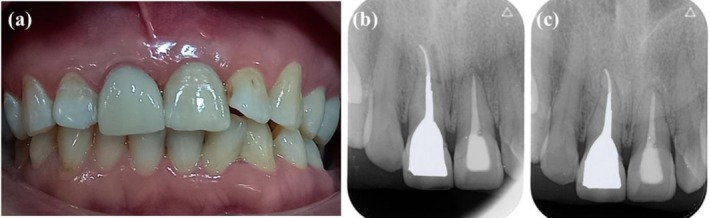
(a) Postoperative clinical photography of a permanent restoration. (b) 1‐month follow‐up radiograph after permanent restoration. (c) 12‐months follow‐up radiograph showing periapical healing.
